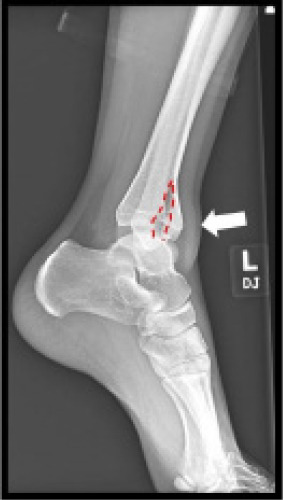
Lateral view plain film reveals a vertical fracture of the distal tibia (red dashed line) and soft-tissue swelling (white arrow).
